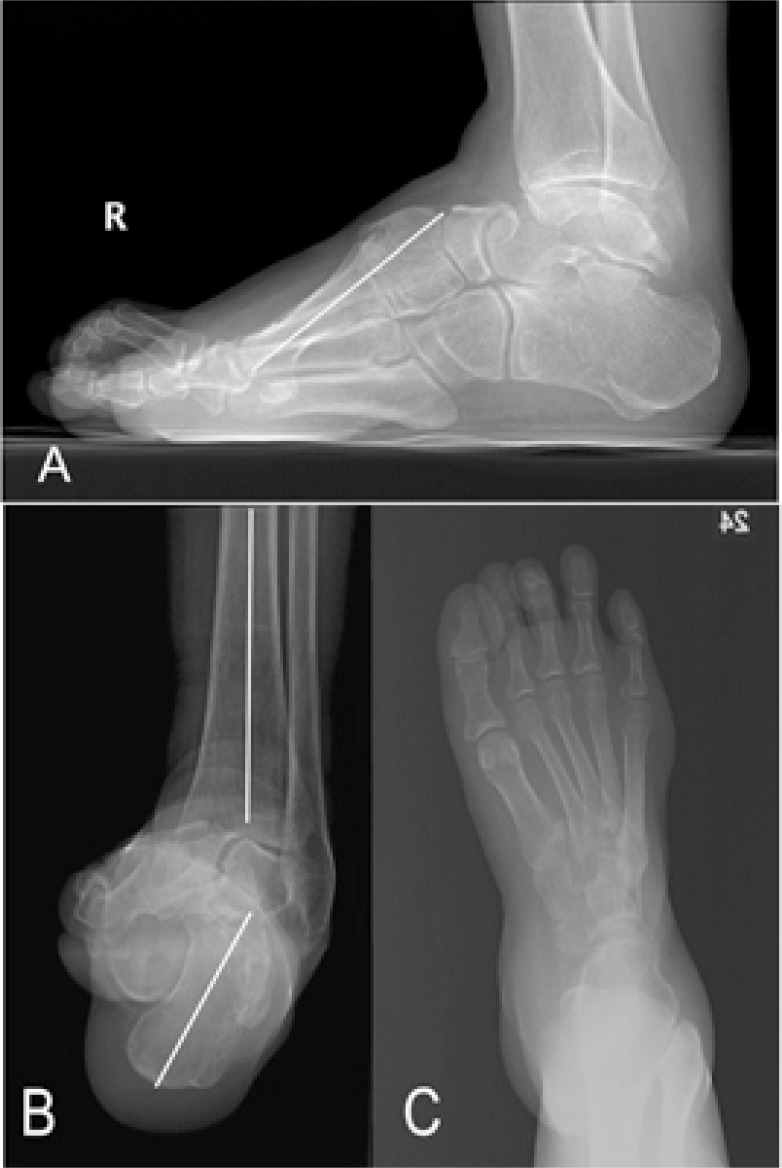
Radiographic findings of a patient with severe cavovarus foot. A standing lateral view (A) shows a plantar flexed 1^st^ ray, elevated navicular height, depressed 5^th^ metatarsal base, and claw toe deformities. Exact Meary angle can not be measured due to severe tilting of the talus. The posterior facet of the subtalar joint as well as the Chopart joints are clearly viewed in this lateral radiograph due to rotation of the hindfoot. The distal fibular is enlarged and posteriorly located. Secondary osteoarthritis of the ankle joint is noted. A heel-alignment view (B) confirms the degree of heel varus. From the dorsoplantar foot X-ray, the metatarsals are adducted and the 1^st^ metatarsal appears short due to plantar flexion.
